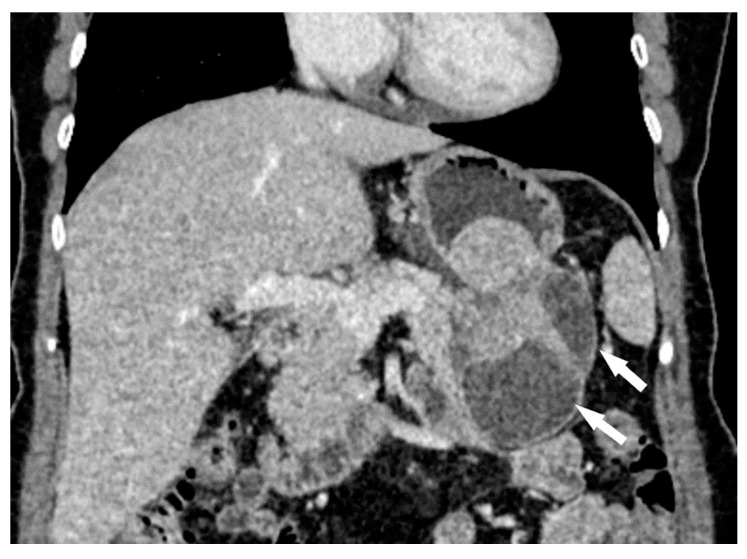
Abdominal CT exam coronal view shows irregular tumor shape with presence of cystic structural changes (white arrow). The tumor corresponds to HR GIST.
